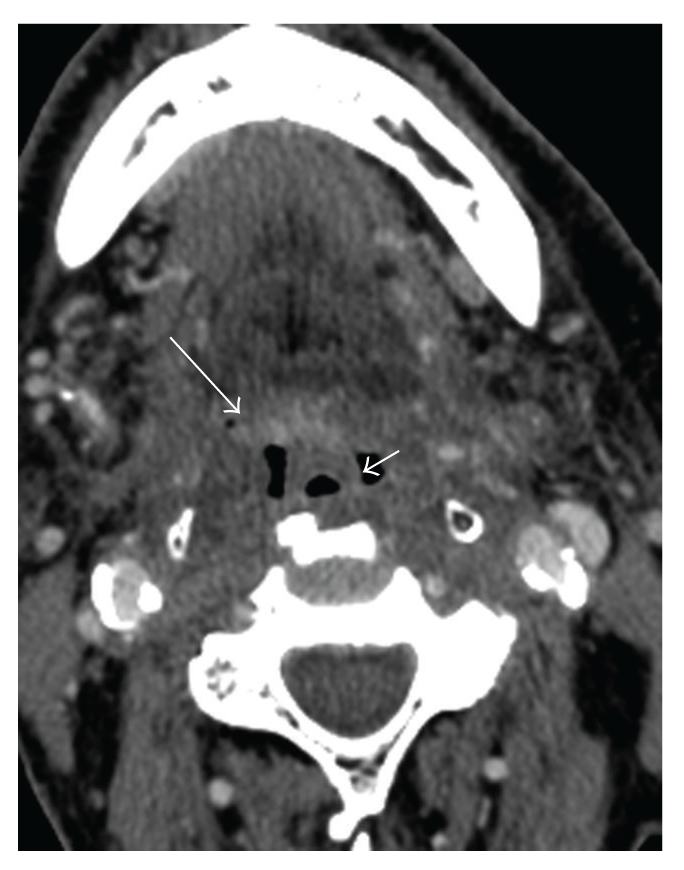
Postradiation changes of the oropharynx: Axial postcontrast CT demonstrates mucositis of the oropharynx characterized by enhancement (large arrow), and edema/swelling, or the epiglottis (small arrow).
